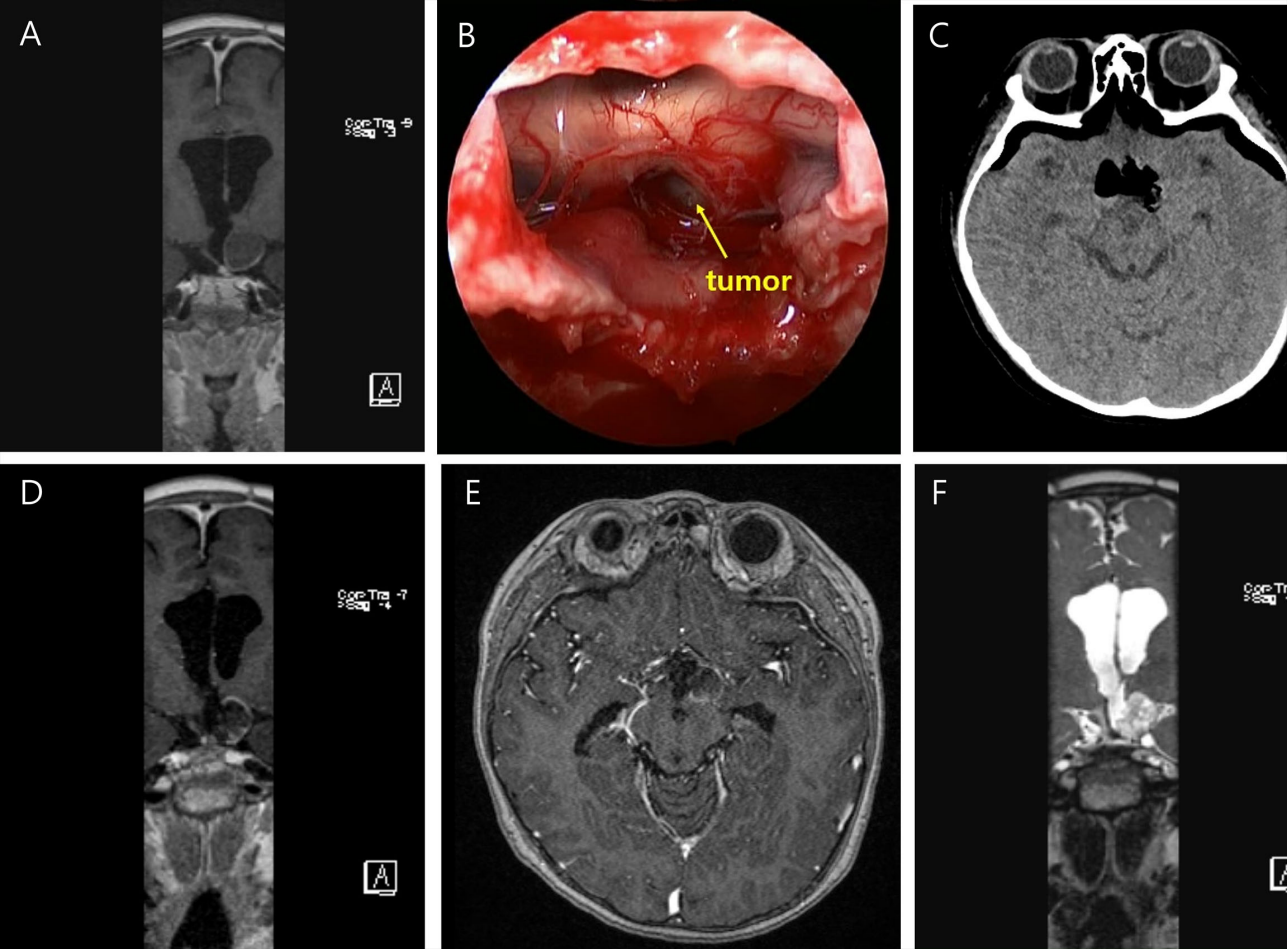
Preoperative T-1 enhanced coronal image of a six year-old male who underwent ESS for recurred craniopharyngioma (A). The tumor is visualized under optic chiasm (B). Immediate postoperative brain CT shows no abnormal finding (C). Postoperative T-1 enhanced coronal (D), axial (E), and T-2 coronal (F) MRI taken within 48 hours after surgery showed no abnormal signal intensities.
